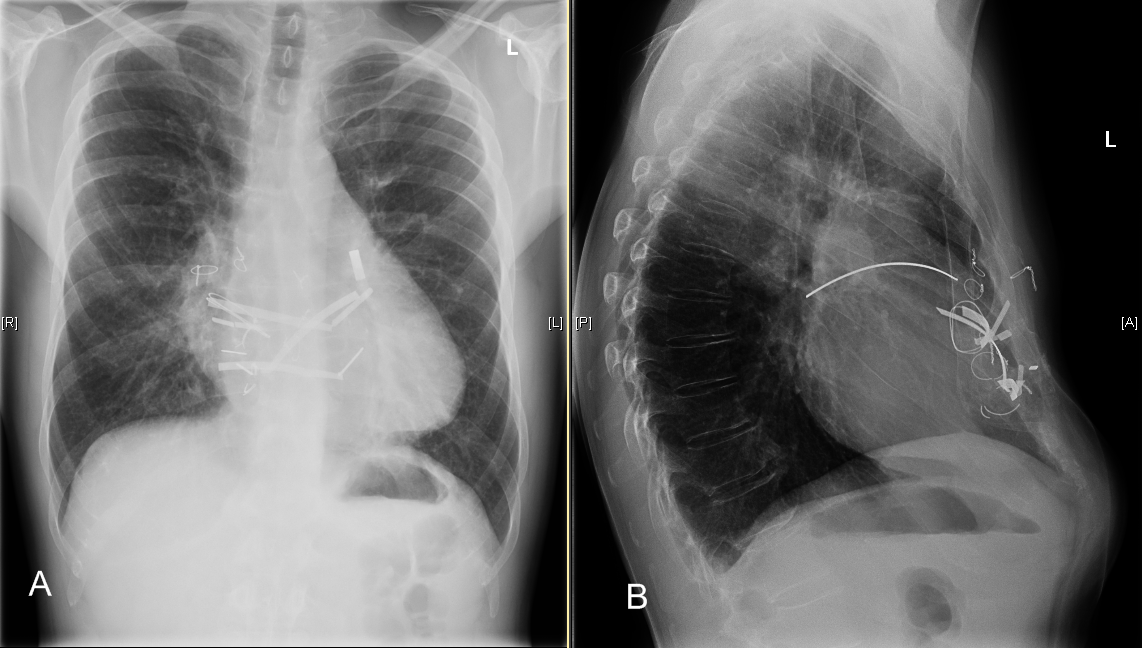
The preoperative chest radiograph. A: The chest radiograph demonstrated multiple dislodged steel struts and wires in a patient, who underwent Ravitch repair for pectus excavatum deformity 37 years ago. B: In the lateral view, several struts seemed to lie in the middle mediastinum.
